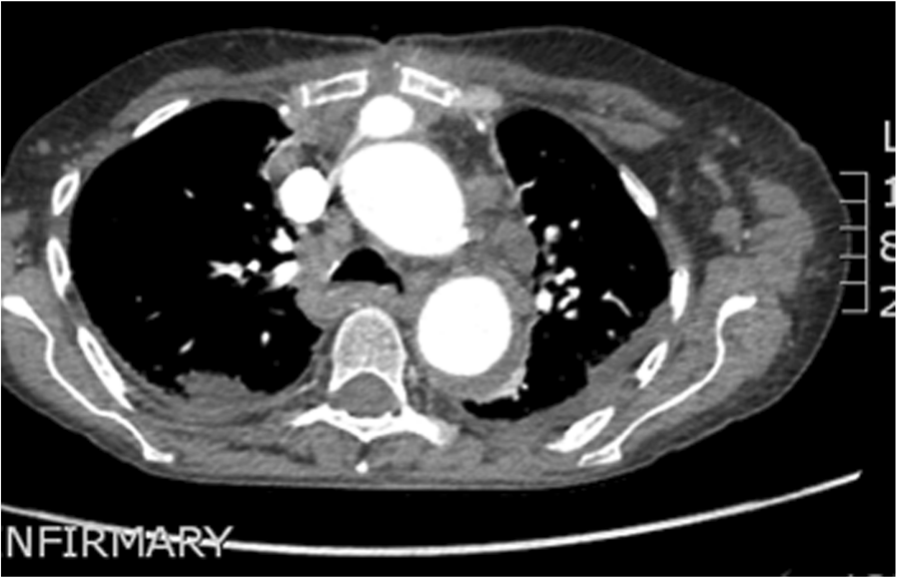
Post-operative CT scan showing old intra-mural hematoma in descending thoracic aorta.
